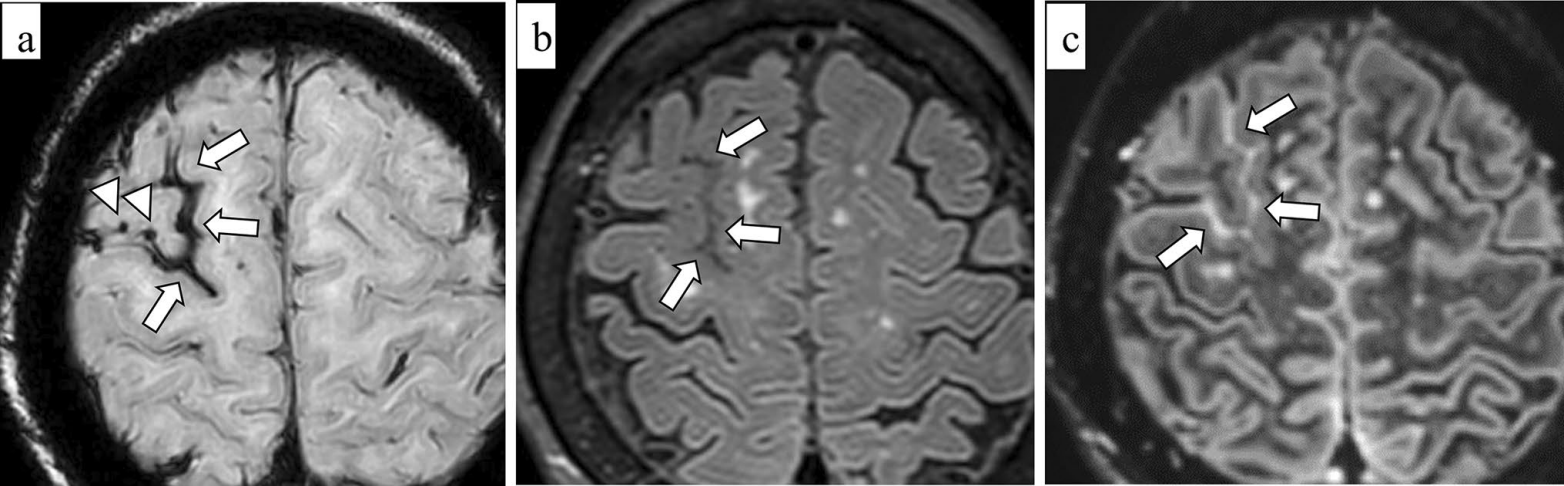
A 71-year-old female with probable CAA and mild cognitive impairment. She presents with headache and dementia with an MMSE score of 24. SWI (a) detects cSS in the sulci of the right frontal lobe (arrows) and lobar CMBs in the adjacent cortex (arrowheads). cSS is more clearly depicted on double-inversion recovery (DIR) (c) (arrows) than 3D-FLAIR (b) (arrows)
